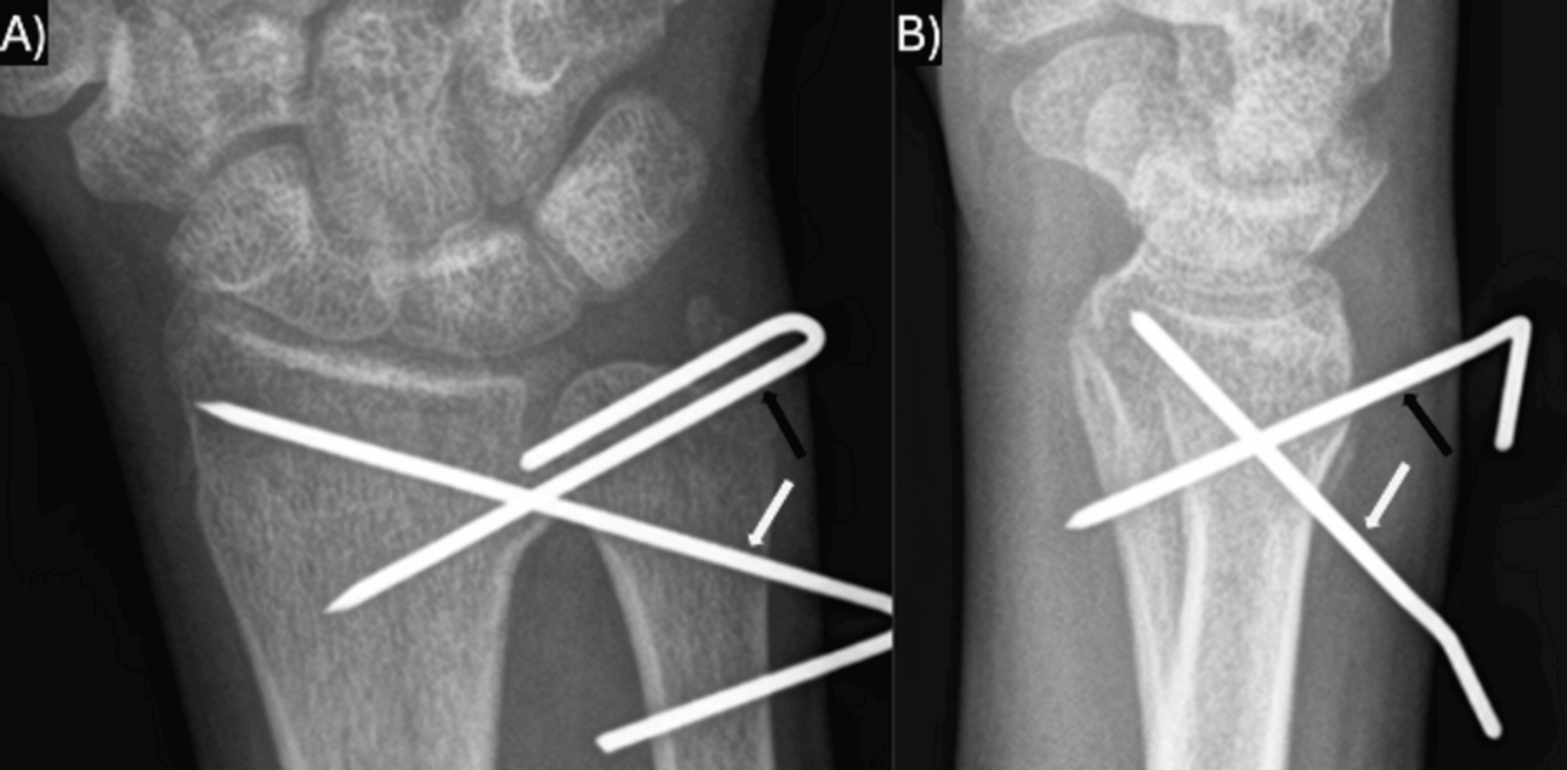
Pinning construct from after CRPP of the operatively managed case V; this case required pinning due to loss of reduction after splinting. Pins were removed at six weeks post-operatively in the clinic after the fracture was demonstrated to be healed. A) AP radiographs at six weeks postoperatively prior to pin removal; B) Lateral radiographs at six weeks postoperatively prior to pin removal. In both panels, the black arrow demonstrates the pin placed dorsally from proximal to distal, while the white arrow demonstrates the pin placed dorsally from distal to proximal. CRPP: closed reduction and percutaneous pinning; AP: anterior-posterior
